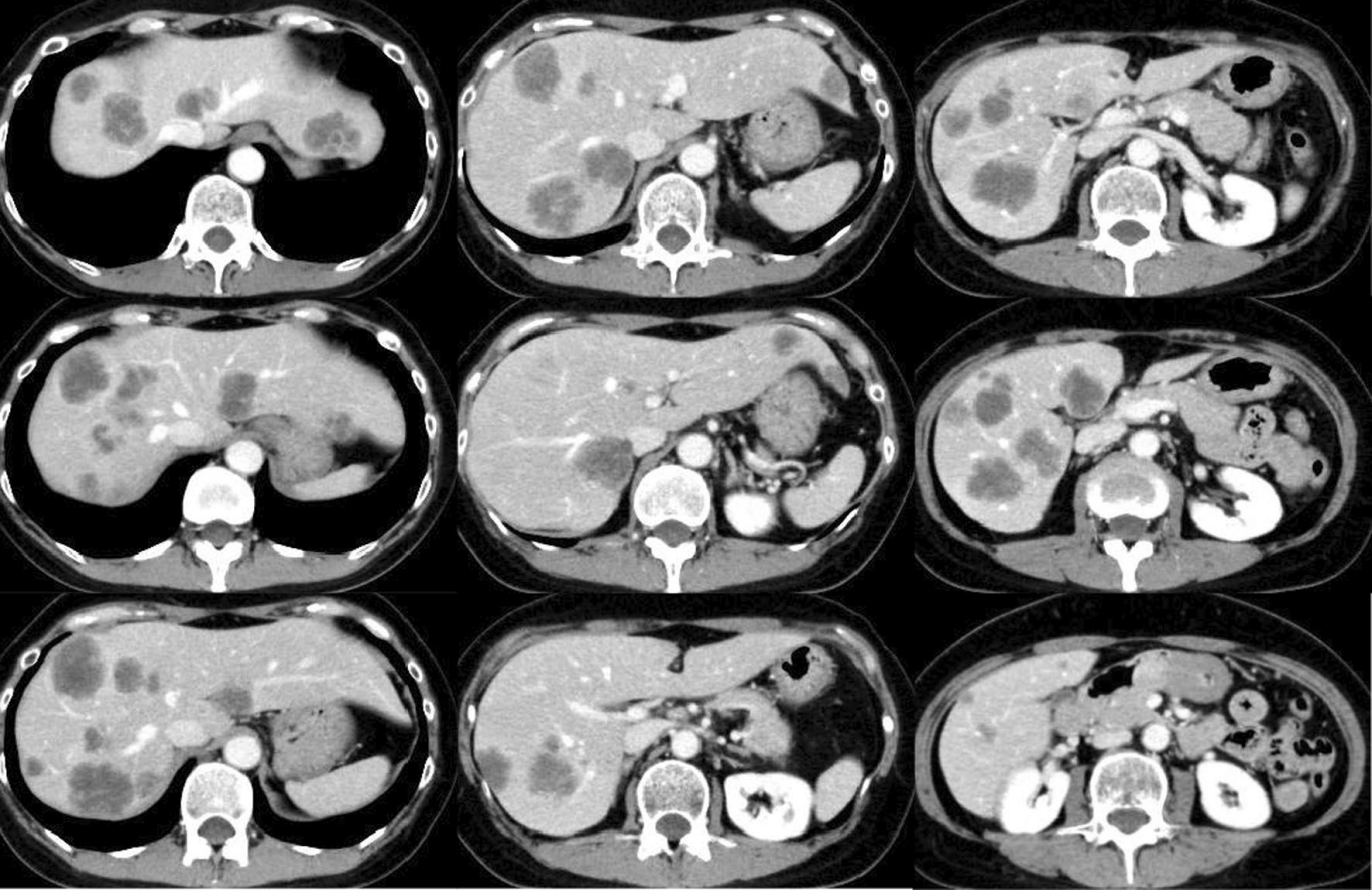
Computed tomographic findings before chemotherapy. Multiple metastases diffusely involve the liver
